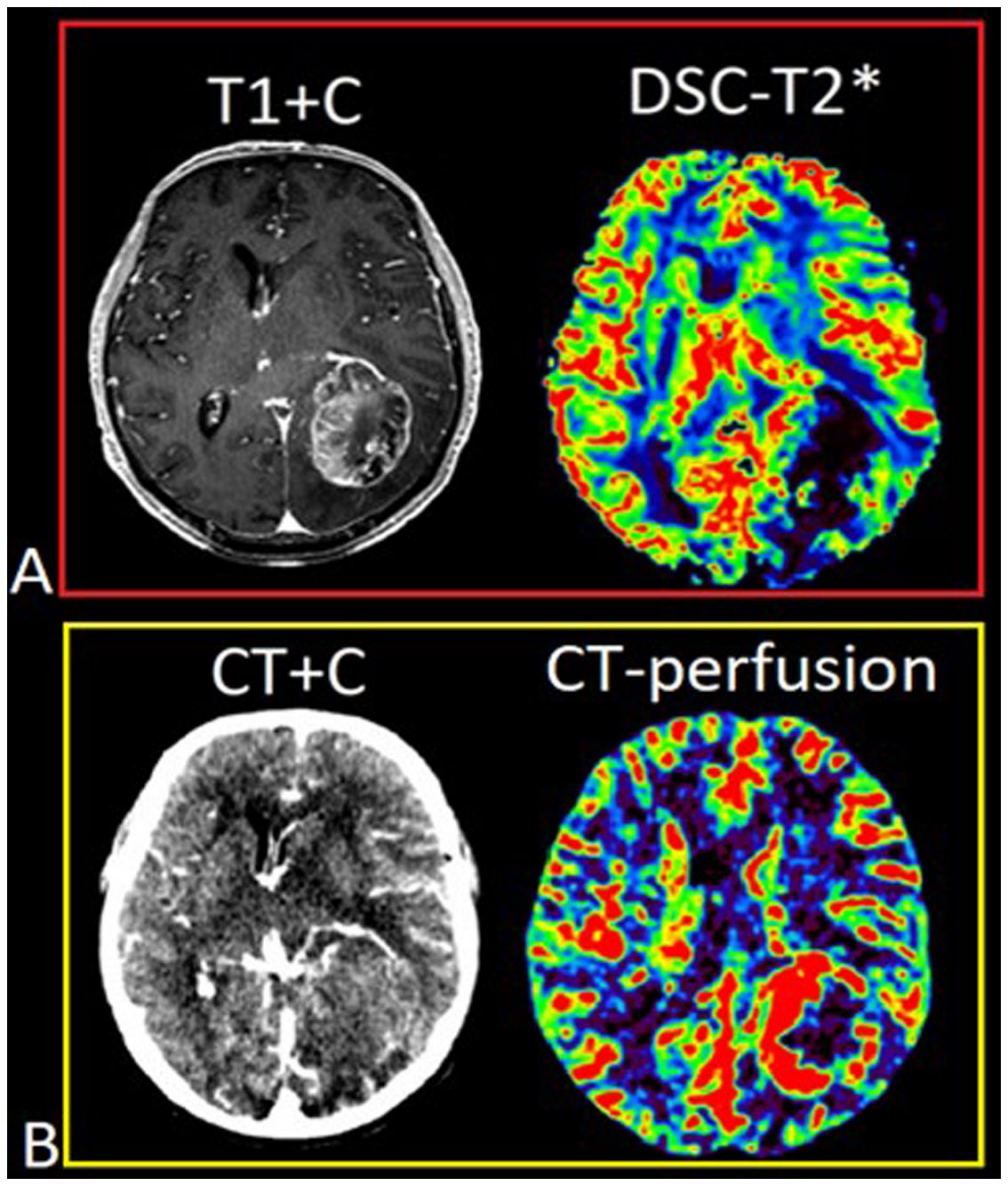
Patient T., 42 y.o., left occipital lobe glioblastoma. (A) Post-contrast T1 WI and DSC-T2* perfusion. DSC-T2* perfusion demonstrates marked magnetic susceptibility artifacts with local signal loss due to blood degradation products. (В) CT with contrast (arterial phase) and CT-perfusion. CT-perfusion does not contain any artifacts and fully displays the tumor boundaries.
